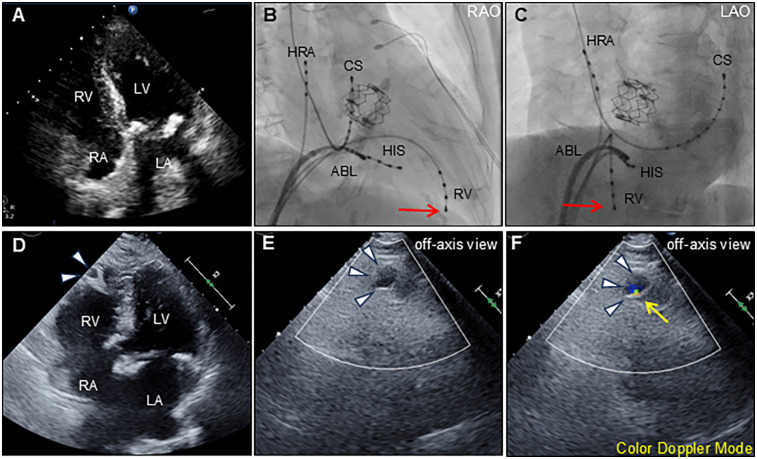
Transthoracic echocardiography and ablation images. Preprocedural transthoracic echocardiography (apical 4-chamber view) shows normal biventricular function with normal anatomy (A) Radiographs of the right anterior oblique [RAO; (B)] and left anterior oblique [LAO; (C)] views show the radiofrequency ablation catheter (ABL) positioned at the posterior right atrial septum. Other catheters were positioned at the high right atrium (HRA), His-bundle (HIS), coronary sinus (CS), and right ventricle (RV). Red arrows indicate the tip of the indwelling RV catheter. Postprocedural transthoracic echocardiography (apical 4-chamber view) reveals an outpouching of the right ventricular apex (arrowheads) (D) Color Doppler echocardiography using an off-axis view reveals outpouching of the right ventricular apex (arrowheads) during diastole (E) and systole (F) The expansion with the antegrade flow at systole, suggests communication between the outpouching and right ventricle (yellow arrow). LA, left atrium; LV, left ventricle; RA, right atrium; RV, right ventricle.
